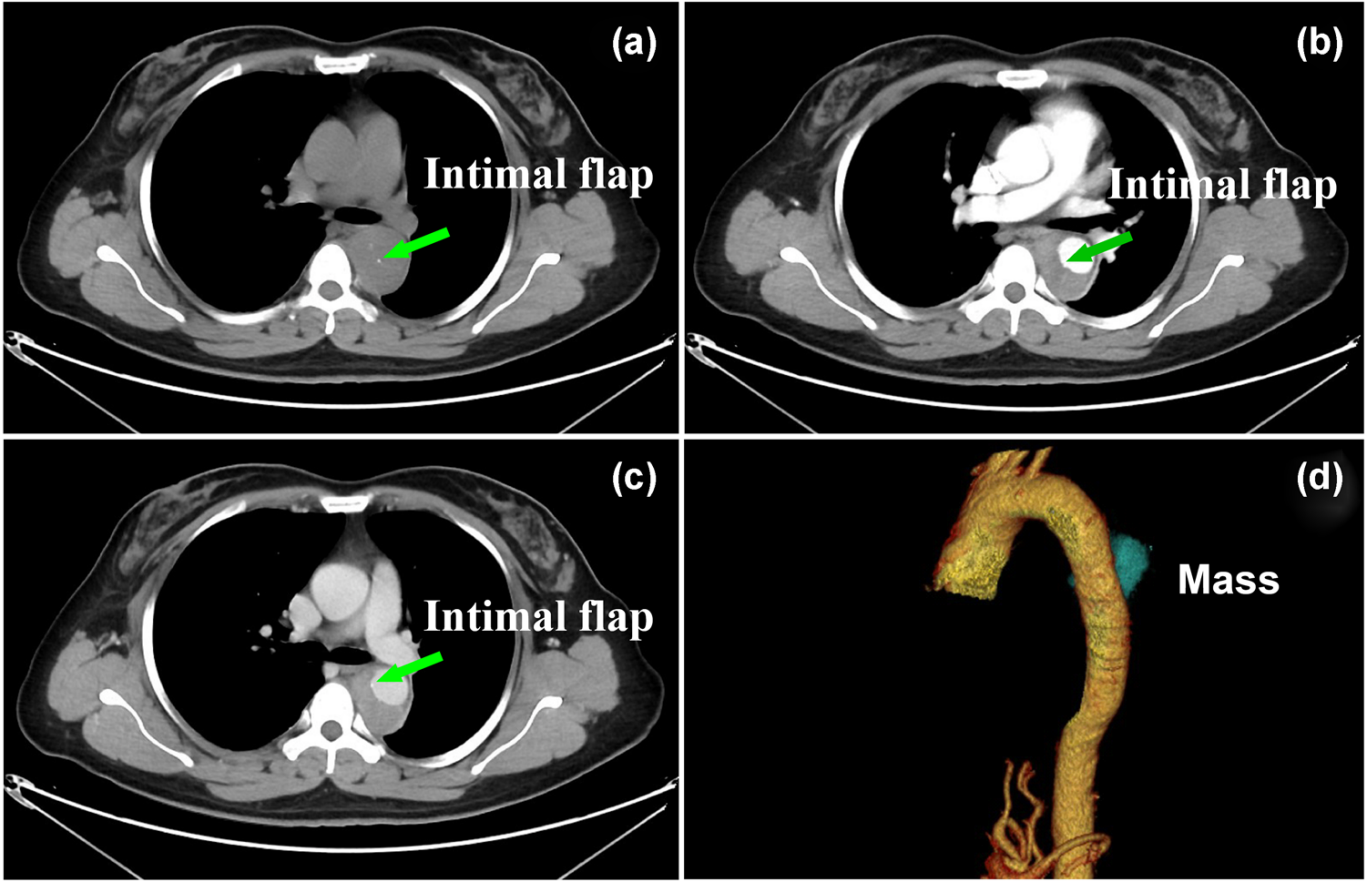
Axial nonenhanced CT images (a) showing a crescent-shaped thickening of aortic wall with the same attenuation as that of the lumen and intimal flap. CTA (c and d) showing an intimal flap and expanded false lumen with slight enhancement, and correspond reconstructed image (d) showing a mass-like false lumen, which was interpreted by radiologists as IMH.
